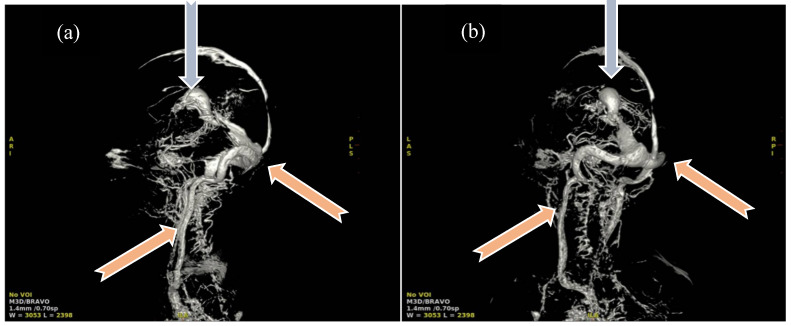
Three-dimensional reconstruction angio-MRI: (a) Sagittal view of the VoGM, (b) posteroanterior view in coronal plane. Aneurysmal median prosecephalic vein drained by the right sinus (blue arrows). Transverse/sigmoid sinuses and internal jugular veins of increased caliber (pink arrows). Multiple vascular pathways at the level of the basal nuclei connecting the anterior and posterior circulation. Carotid, vertebral arteries and basilar trunk exerting mass effect on the bulb.
